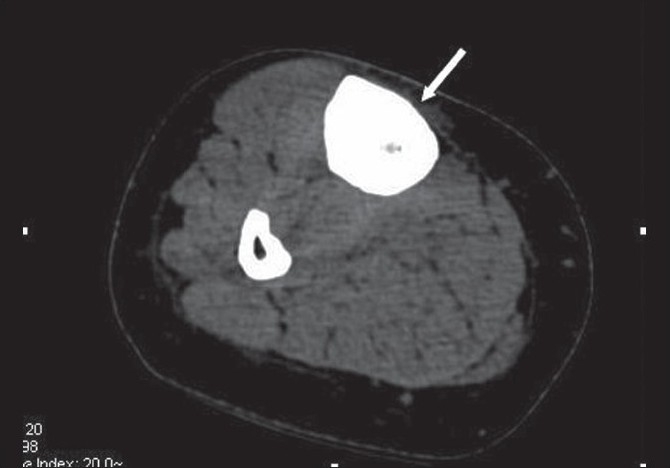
Axial plain CT scan of the right tibia demonstrates severe cortical thickening, with almost complete obliteration of the medullary cavity (arrows)
